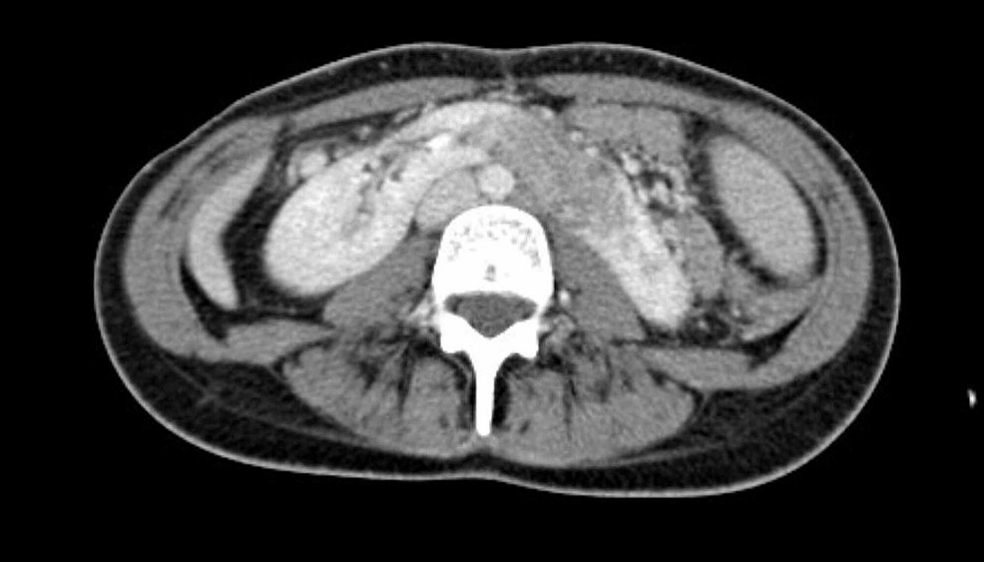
Abdominal CT scan without contrast shows horseshoe-shaped kidneys with suspicion of renal mass.
